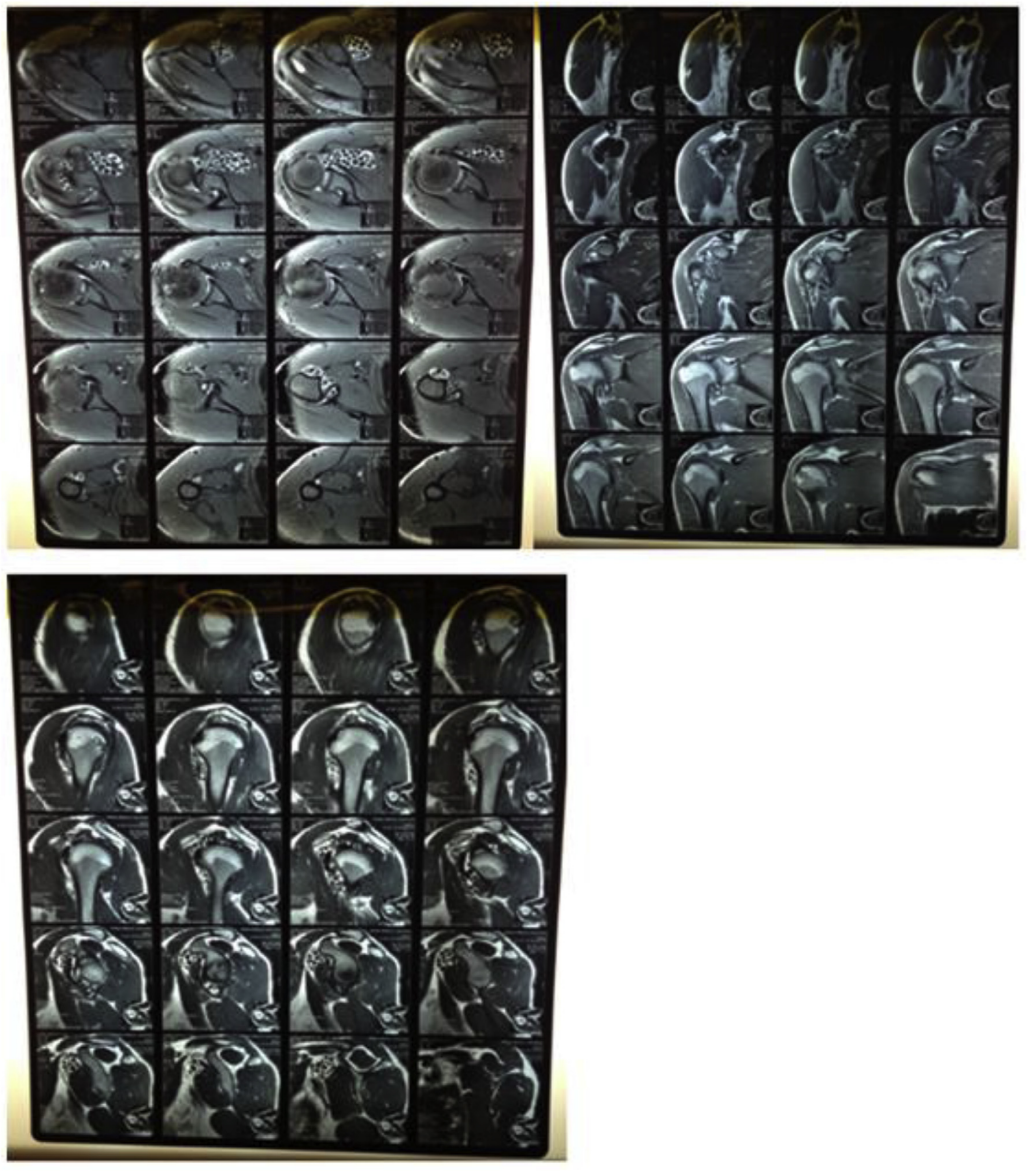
Pre operative MRI was done to know exact location of loose bodies and to look for other pathologies they are loose bodies and synovitis no other pathology or tendenopathy was identified upper left image axial view of shoulder joint showing loose bodies upper right image coronal view of shoulder joint showing loose bodies pre operative MRI sagittal view of shoulder joint showing loose bodies.
